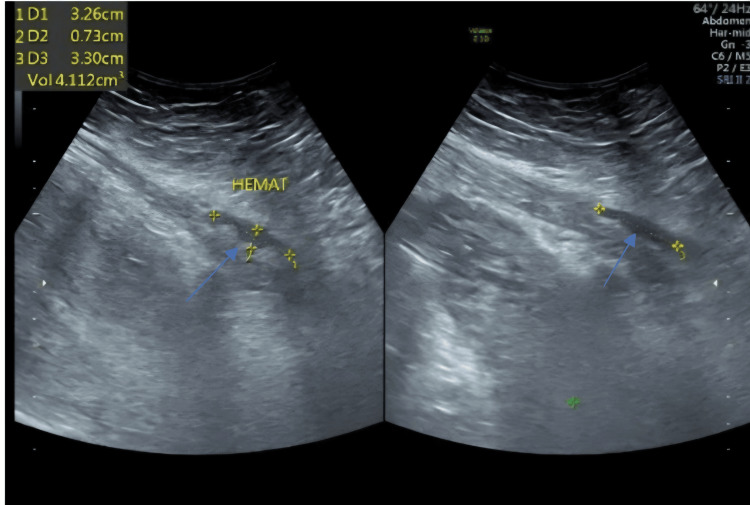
Pelvic ultrasound showing hematoma measuring 33x32x0.73 mm at the surgical site
